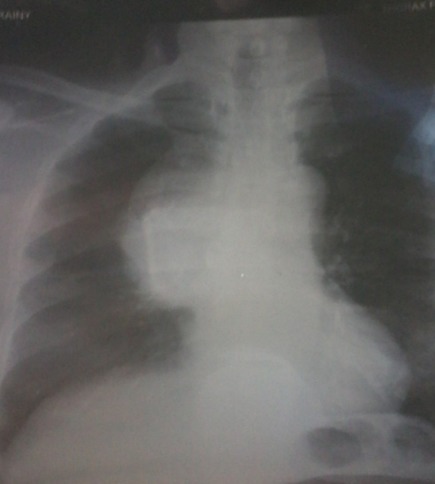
Radiographie du thorax: élargissement du médiastin, IMT 0, 4
